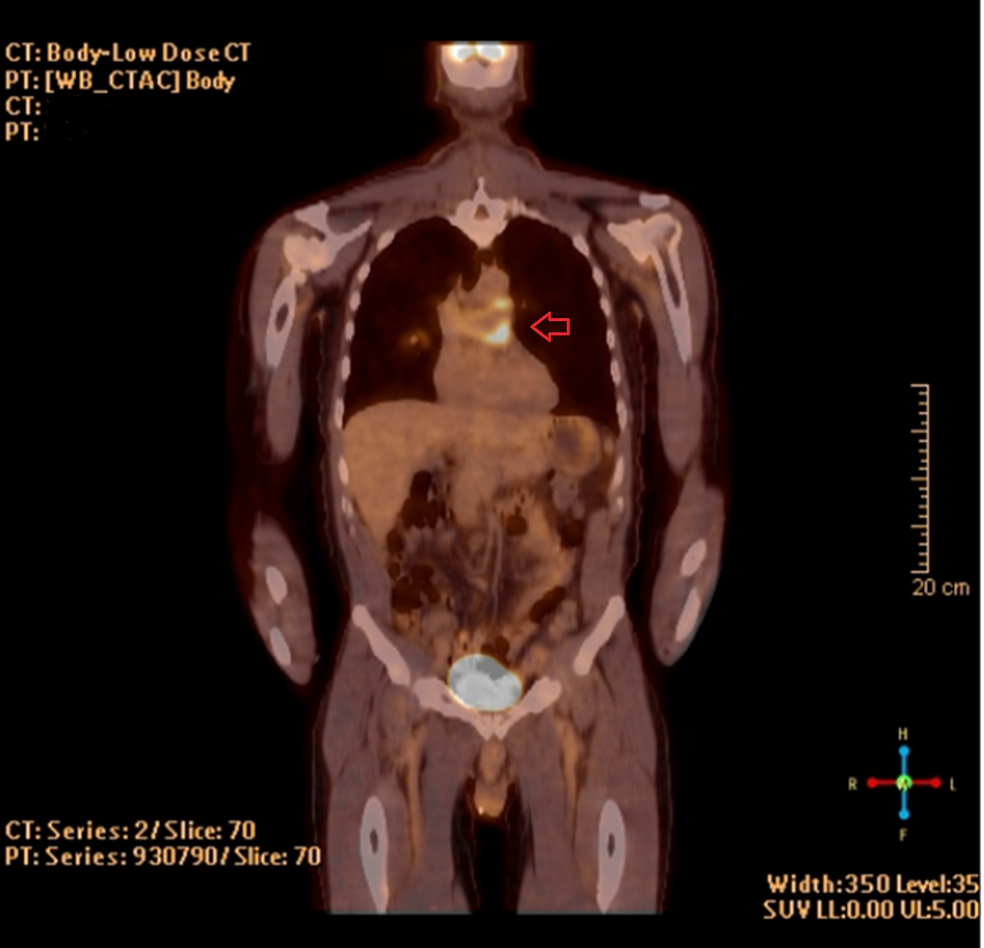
PET/CT demonstrating hypermetabolic lesion within the pulmonary artery and extending bilaterally (arrow) PET: positron emission tomography; CT: computed tomography
